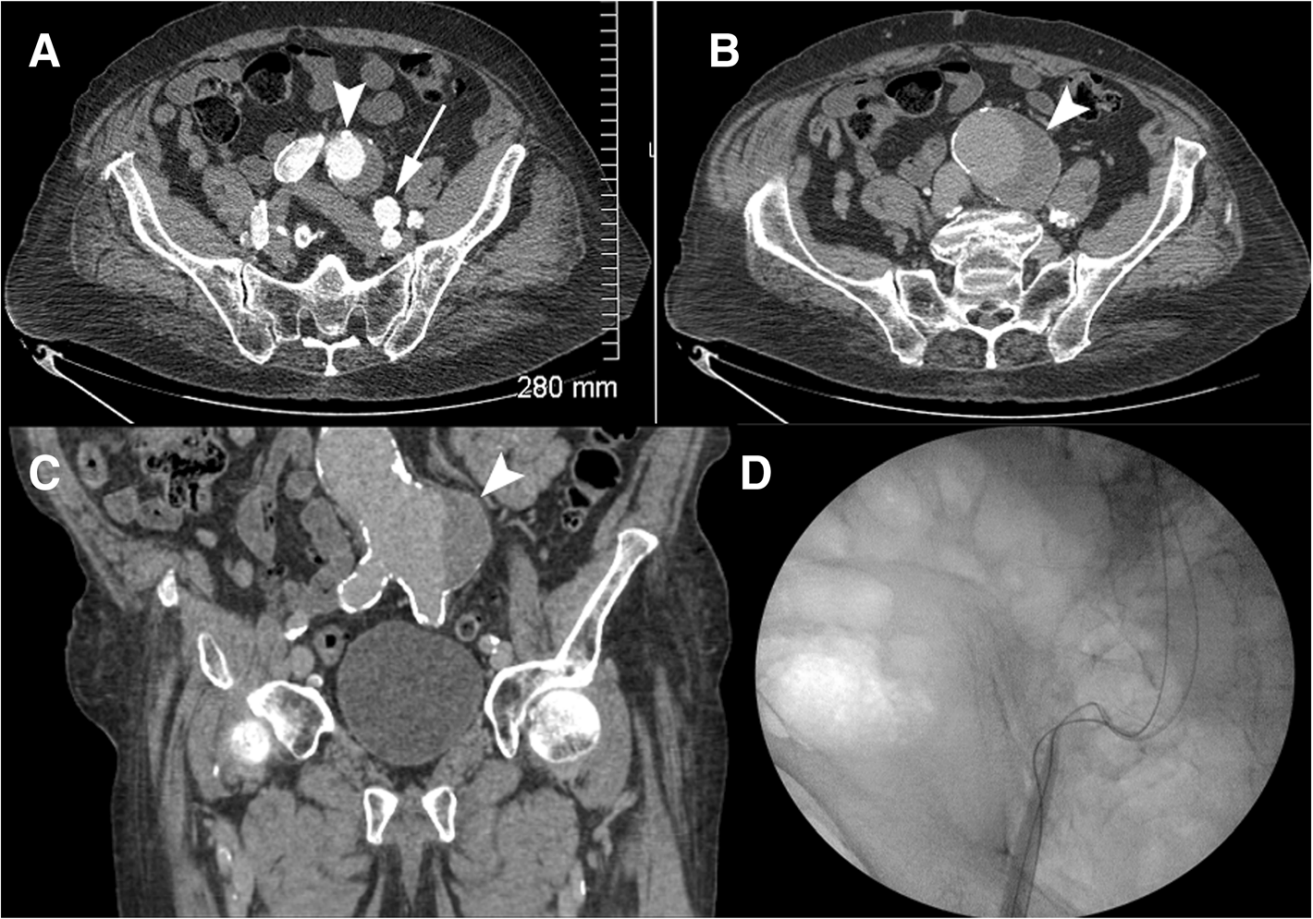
Contrast-enhanced CTA images demonstrate a. tortuosity of the iliac vessels (common iliac, arrowhead; external iliac, arrow) b. abdominal aortic aneurysm (arrowhead). c. Two-dimensional reconstructed sagittal image highlights the infrarenal aortic aneurysm (arrowhead) and iliac and aortic vascular calcifications. d Intraoperative fluoroscopic image of the pelvis during endograft TEVAR attempt demonstrates marked aorto-iliac tortuosity
